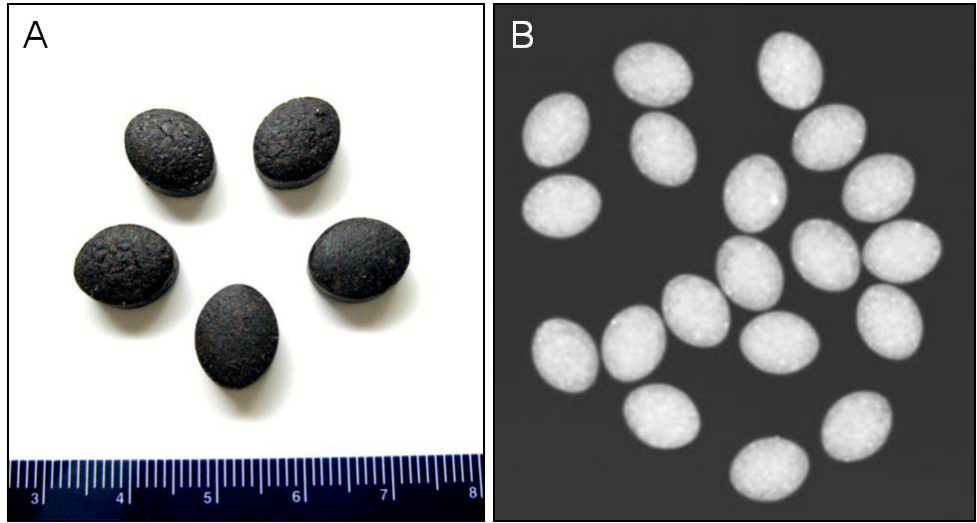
Jambrulin tablets. A. The tablets are 1.25 cm black, ovoid pills (ruler is in cm). B. X-ray shows diffusely opaque Jambrulin pills with flakes of high attenuating material.
